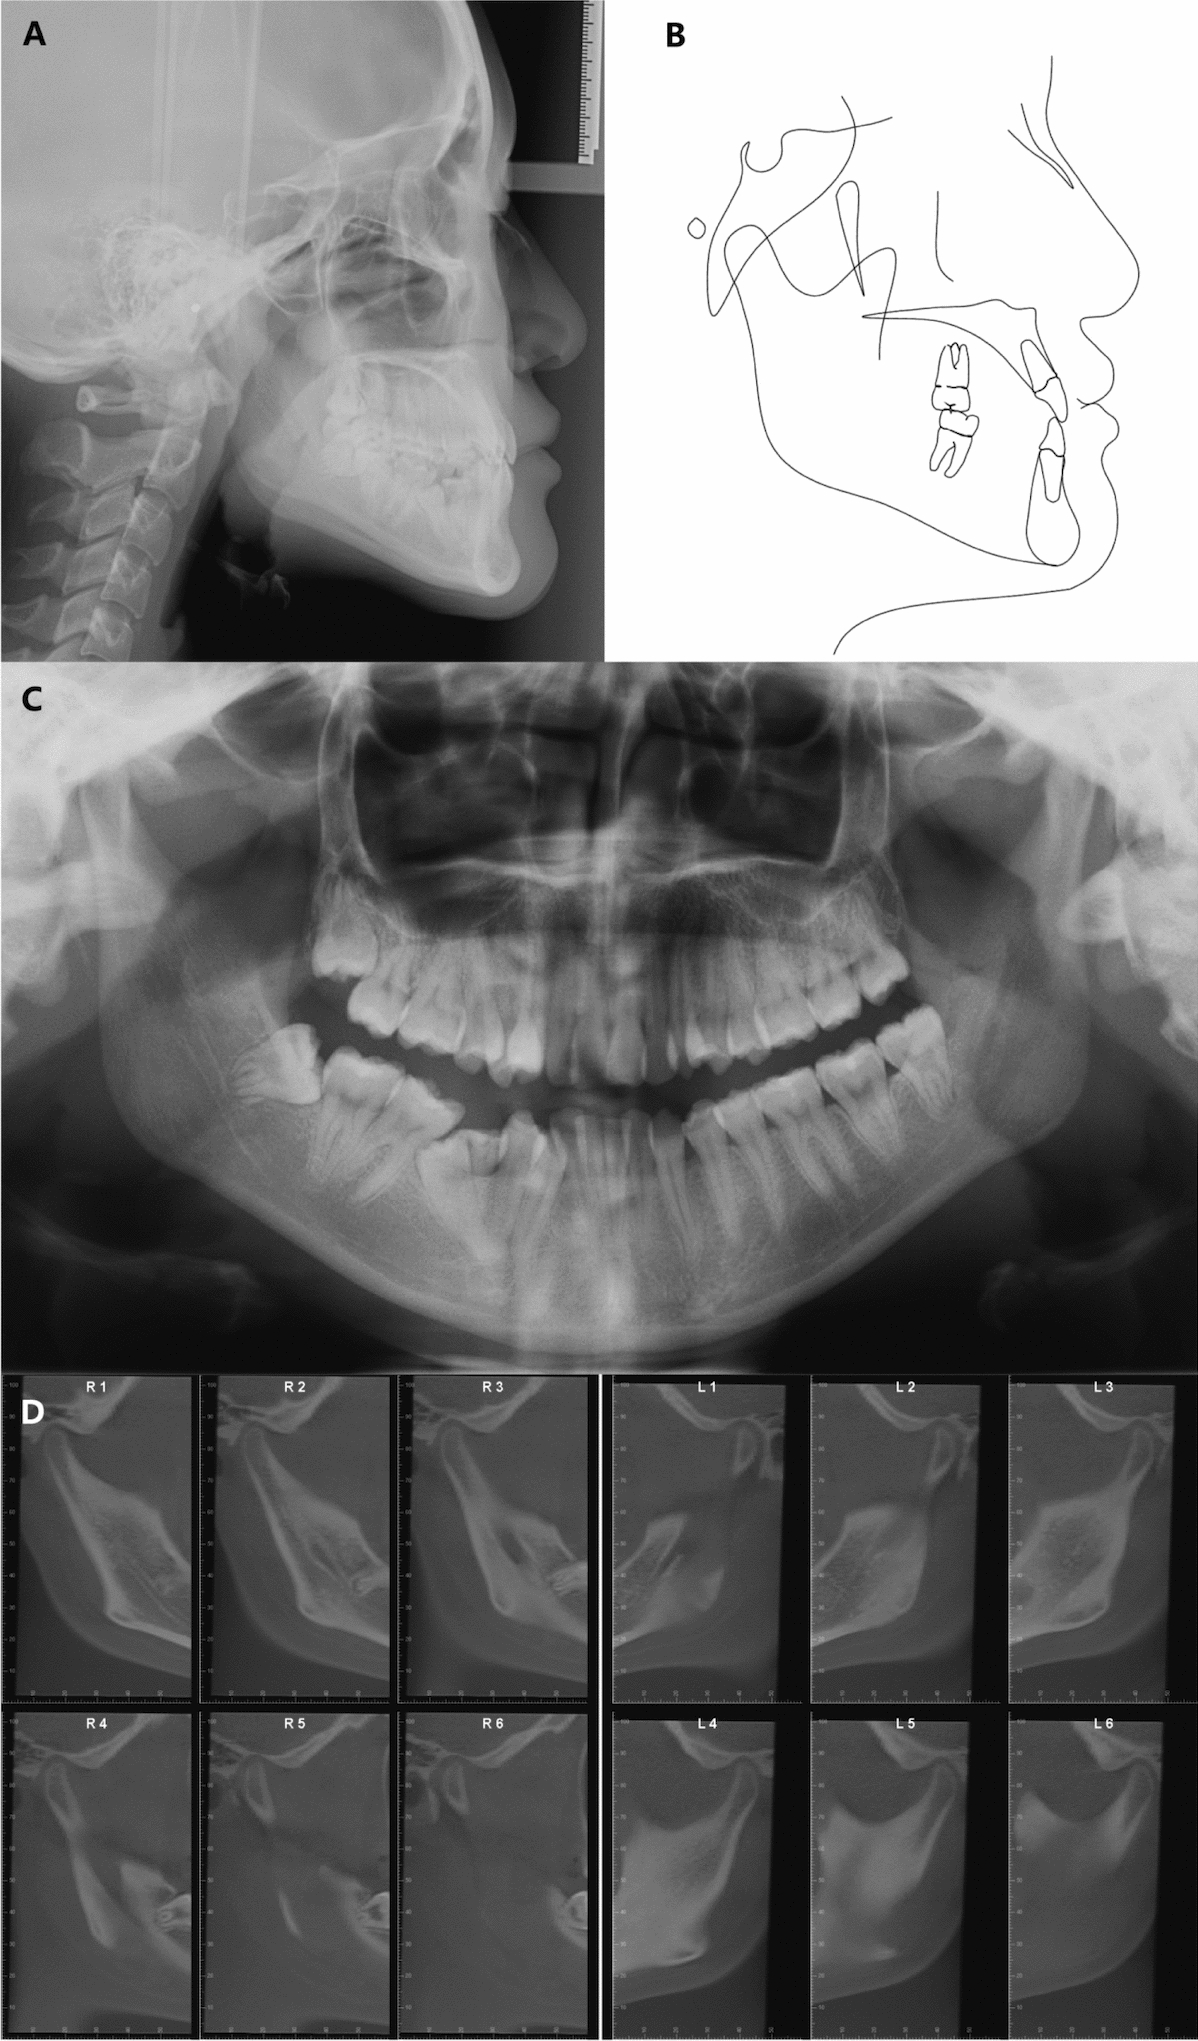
Pretreatment radiographs: A Lateral cephalogram. B Lateral cephalogram tracing. C Panoramic radiograph. D Cone-beam computed tomography image of both TMJs. L, left TMJ; R, right TMJ
